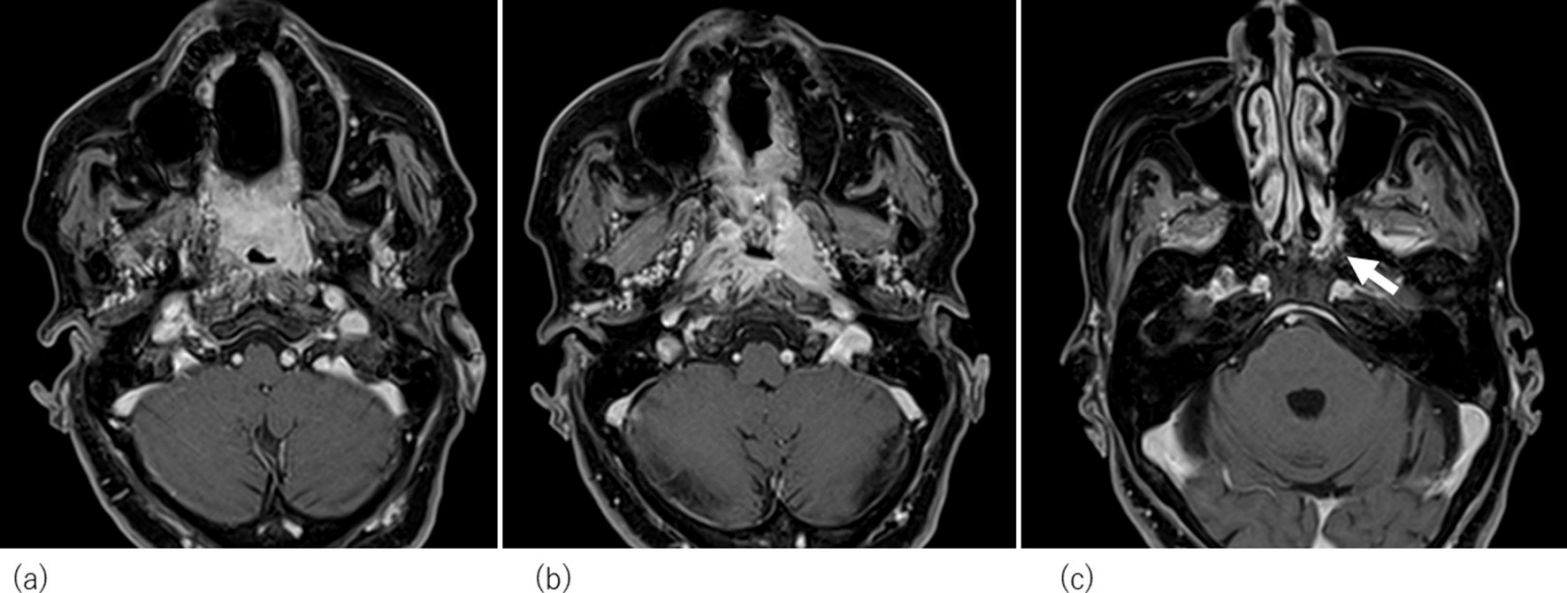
Magnetic resonance images of nasopharyngeal squamous cell carcinoma in the inferior wall of the nasopharynx before treatment. Because left sphenoid bone invasion was found (white arrow in Figure 2 (cFigure)), the tumour was classified as T3.
